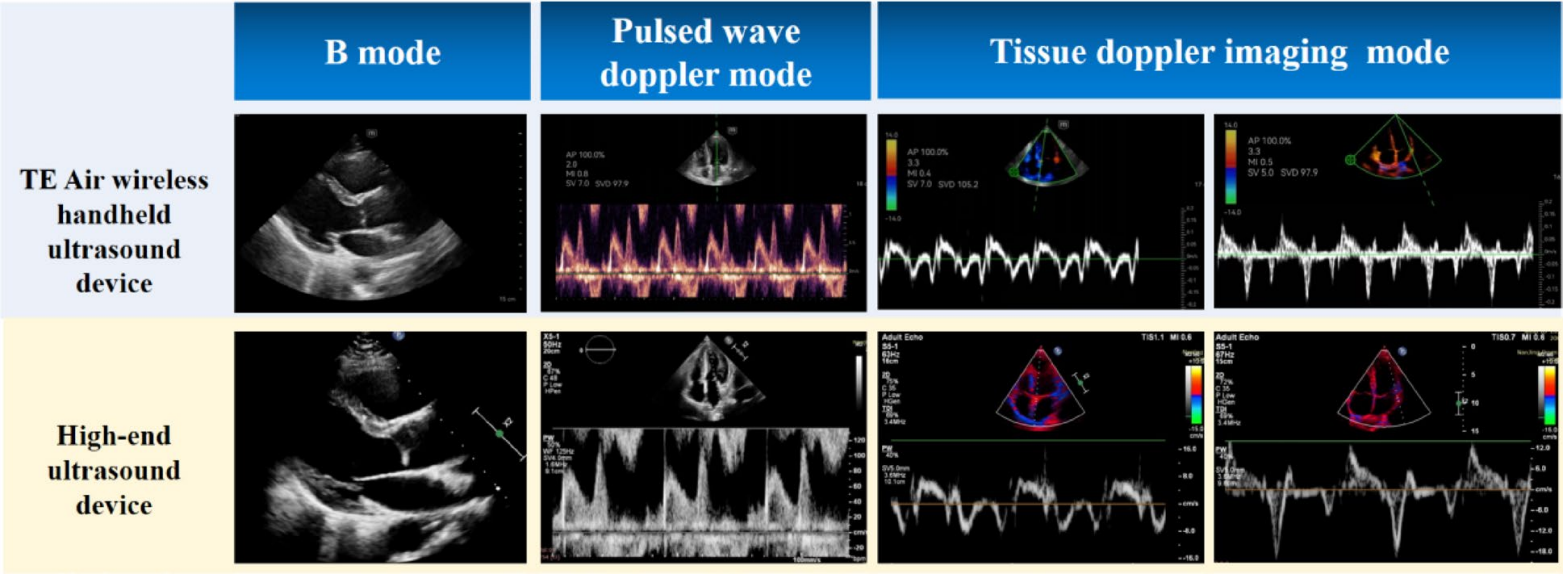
Representative views of patients for B, pulsed wave doppler and tissue doppler imaging
